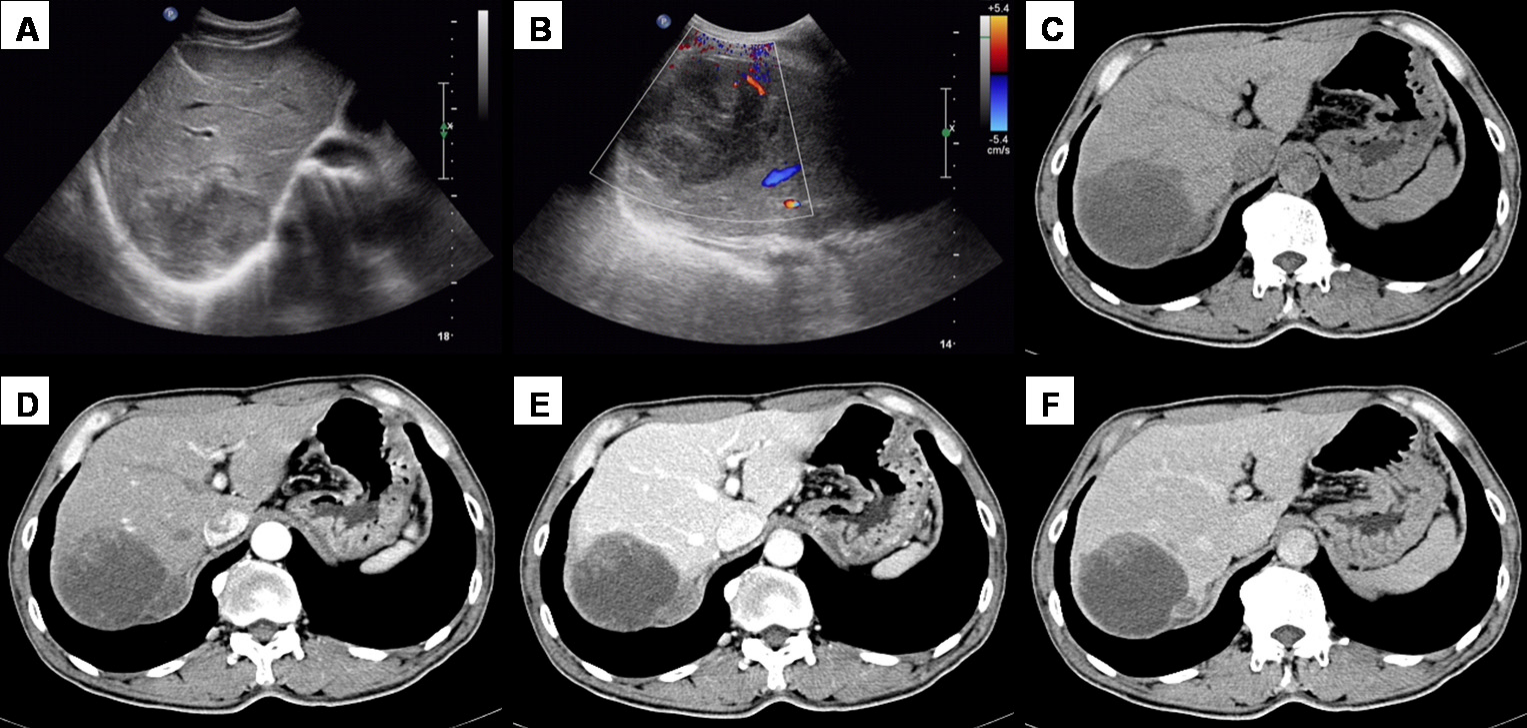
A 56-year-old man with abdominal discomfort. (A, B) Abdominal sonography revealed a hypoechoic mass with low vascularity. (C) Non-enhancement CT showed a heterogeneous hypodense mass with areas of cystic changes in the right lobe. (D- F) After the contrast injection, the mass was slightly enhanced, especially on the edge.
